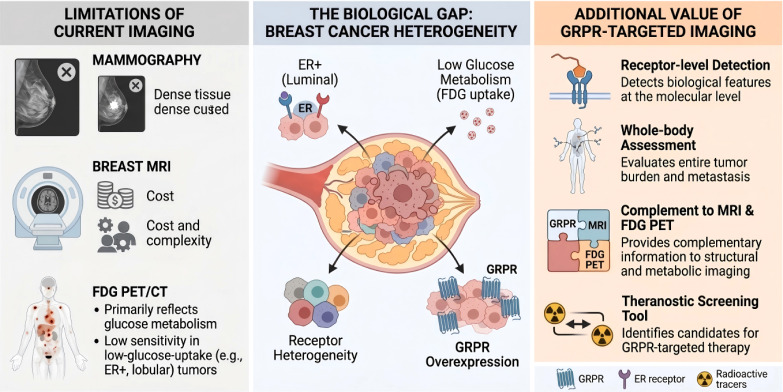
Rationale for GRPR-targeted imaging as a complementary approach in breast cancer. Current imaging modalities in breast cancer remain limited by structural and metabolic constraints, including reduced performance of mammography in dense breasts, the high cost and complexity of MRI, and the low sensitivity of ^18^F-FDG PET/CT in tumors with low glucose metabolism. Given the biological heterogeneity of breast cancer, especially in ER-positive/luminal phenotypes with potential GRPR overexpression, GRPR-targeted imaging may fill this diagnostic gap by providing receptor-based whole-body assessment, complementary information to MRI and FDG PET, and a screening tool for GRPR-targeted theranostic approaches.
